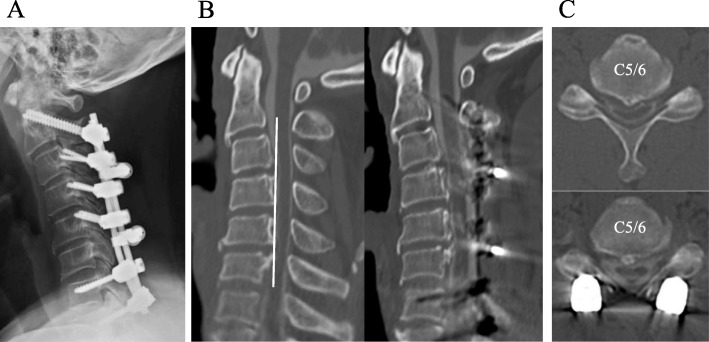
A representative case. a A lateral radiograph after surgery shows posterior decompression and fusion surgery were performed. b A reconstructed sagittal CT myelograph before and 1 year after surgery. The white line represents the K-line. The OPLL in this case is K-line (−). c An axial CT myelograph at the C5/6 level. Sufficient decompression was obtained
